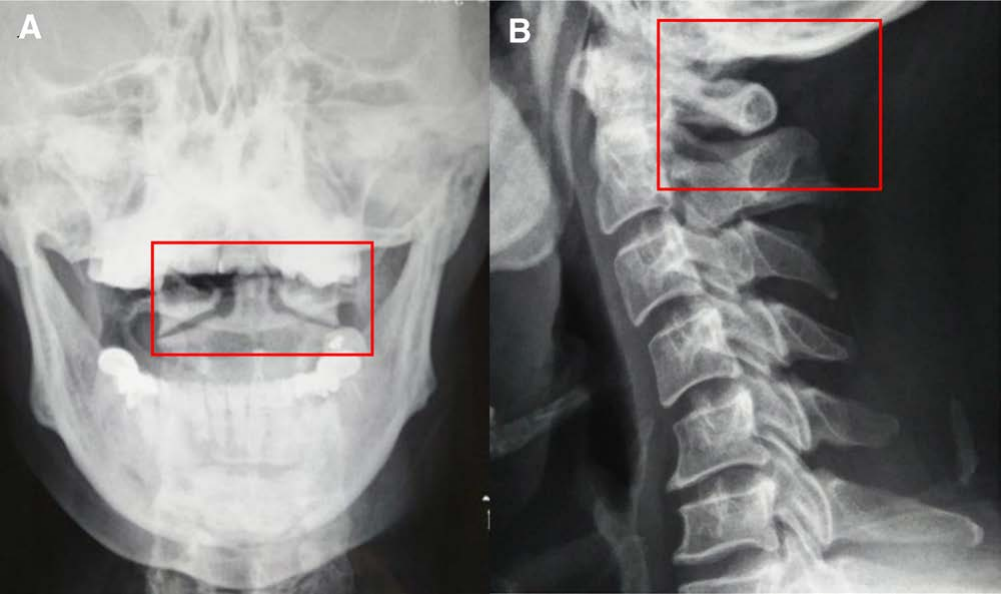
(A) Cervical open mouth X-ray (cervical open mouth X-ray); (B) lateral X-ray of the cervical spine (median sagittal section).
